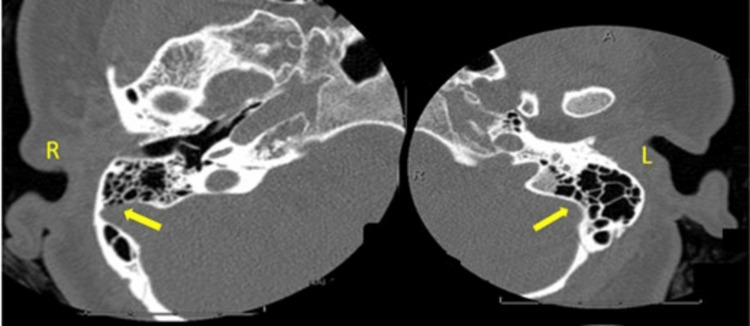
CT axial views revealing thinning, and an area of dehiscence, along the posterior wall of the right mastoid bone in close association with the sigmoid sinus compared to the left (arrows).
